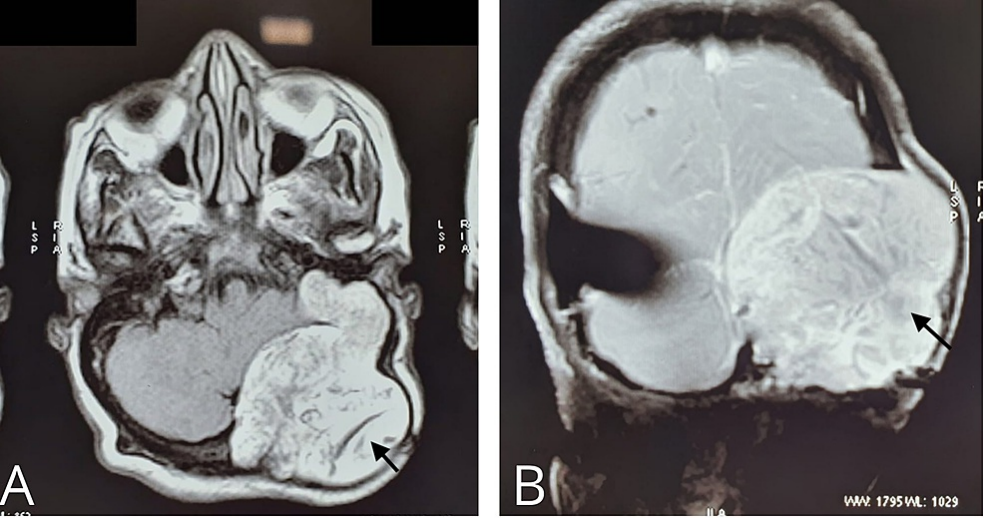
T1-weighted axial (A) and coronal (B) MRI demonstrating a space-occupying lesion with mixed-signal intensities.
